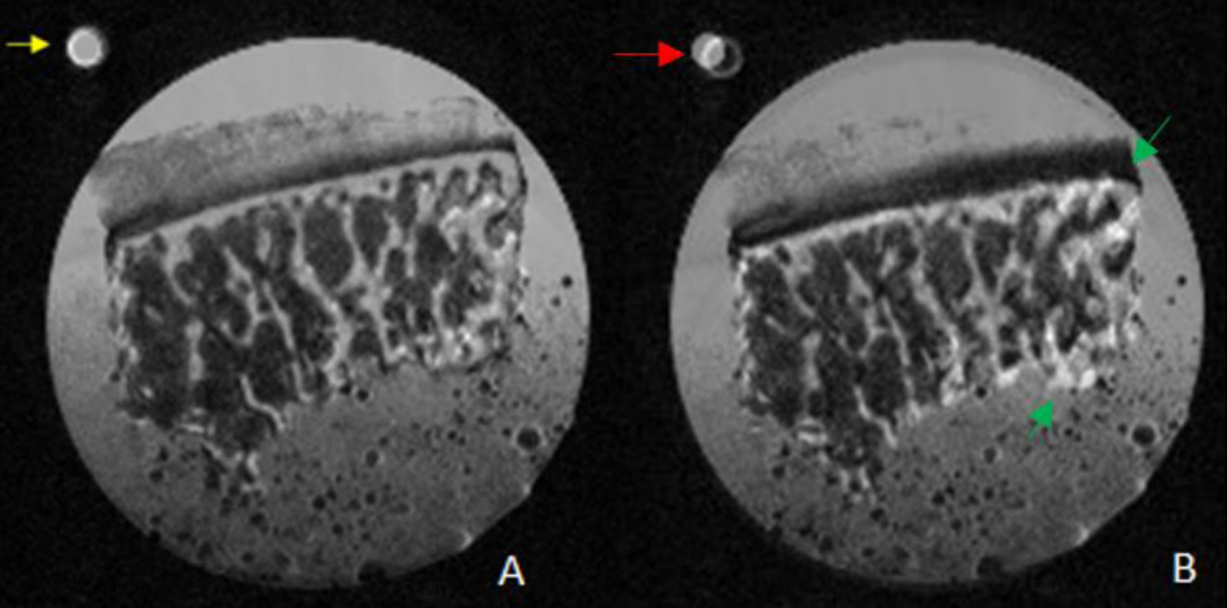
A successful (A) and an unsuccessful (B) first alignment of a micro-magnetic resonance imaging scan and a micro-computed tomography scan of the K-F1 sample. (A) Yellow arrow points to capillaries from both scans, which appear as one single capillary as a result of a successful alignment. (B) Red arrow points to capillaries from both scans, which are not aligned. Green arrows point to the parts of the sample that are protruding as a result of an unsuccessful alignment.
